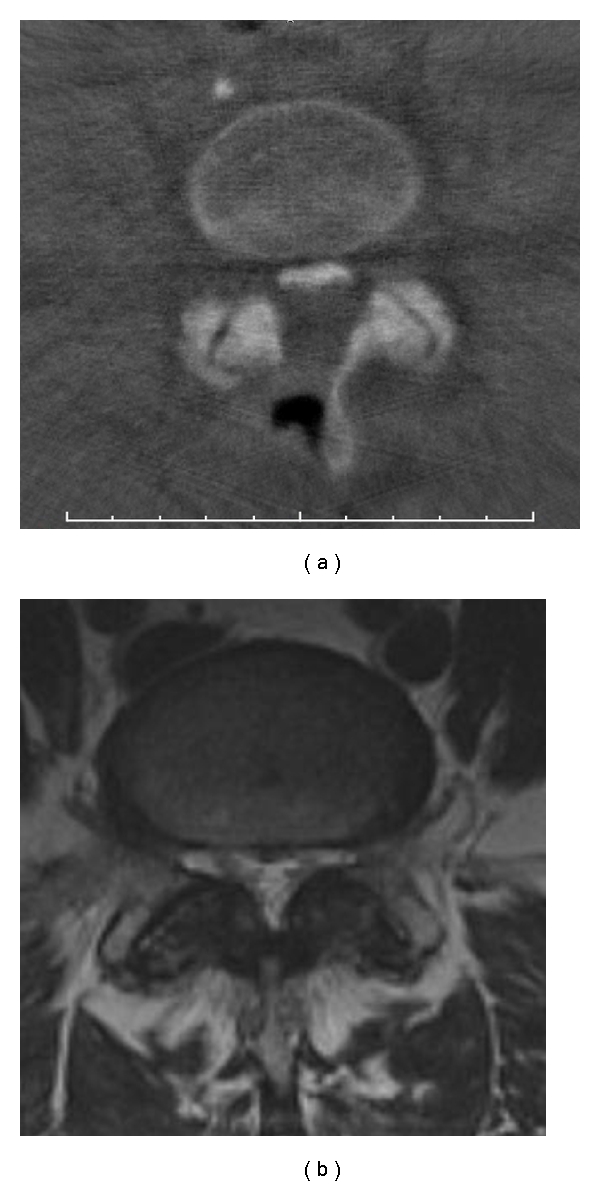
(a) Axial reconstruction demonstrating decompression from the left side with sufficient decompression on the right side. (b) Preoperative MR image of the same patient as in (a).
